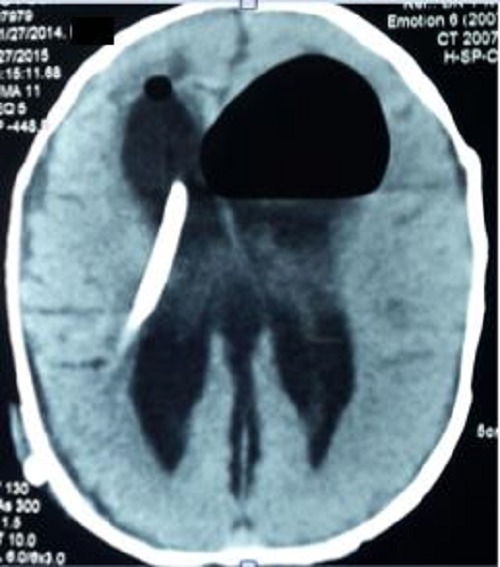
Figure 2: CT head showed air-fluid levels in both ventricles.
